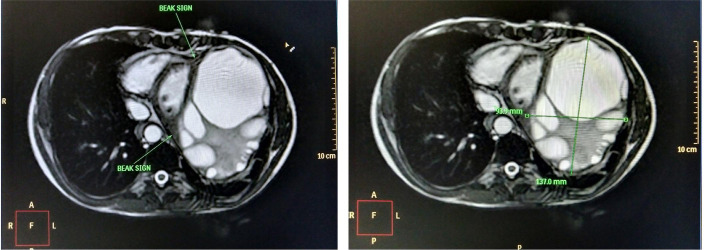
Transverse section on cardiac magnetic resonance imaging showing beak
